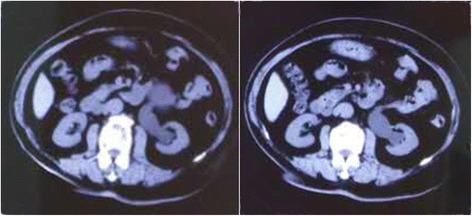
CT:Moderate hydronephrosis
